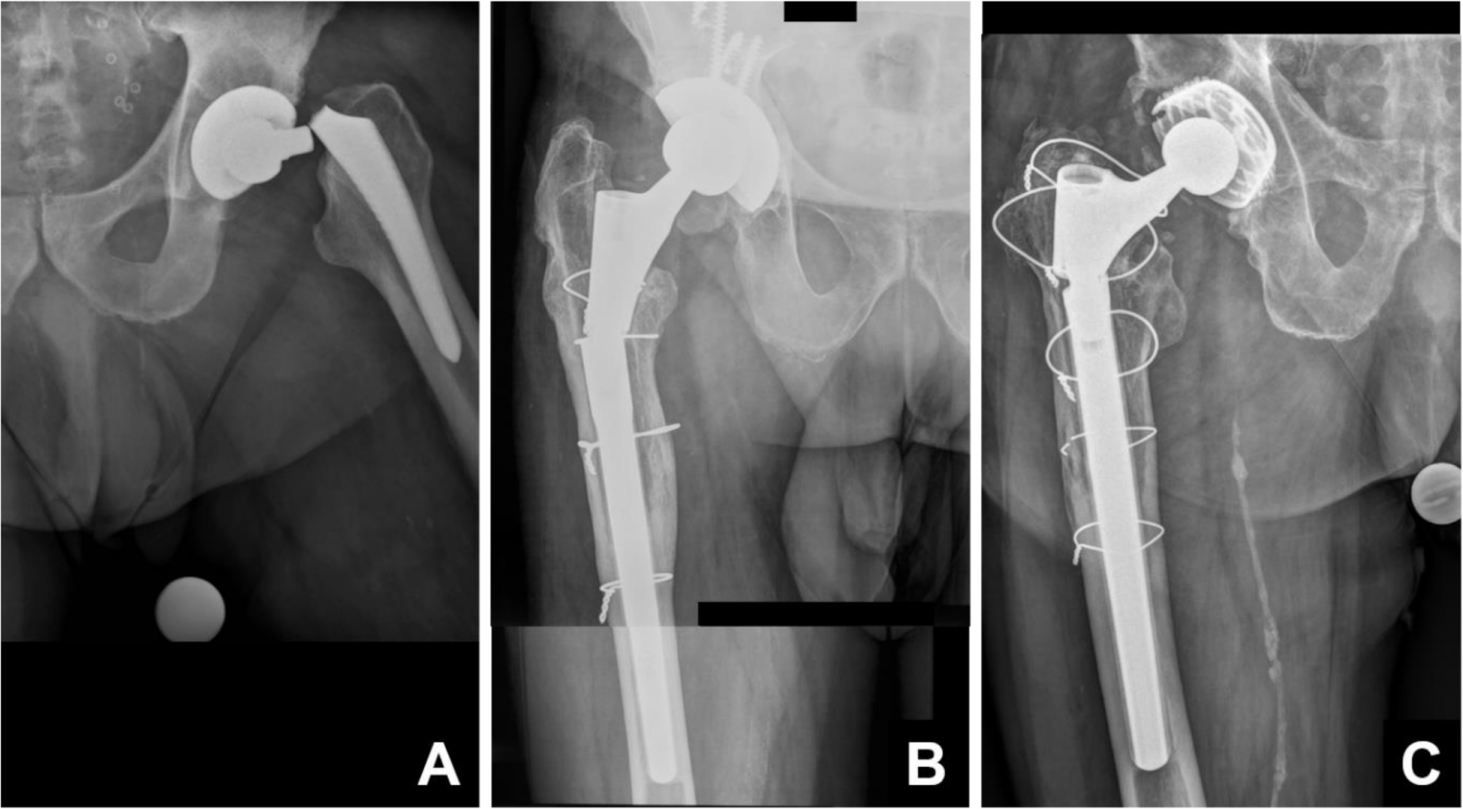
Zone of interest of the anteroposterior pelvic radiographs of three different patients presenting with a well-integrated, broken, uncemented stem after hip arthroplasty. All cases have in common that no standard extraction component can be fitted anymore. In a, a 76-year-old male patient presenting less than 4 years after stem-preserving revision with exchange of the head, performed due to instability, with a well-fixed uncemented primary stem (AMIStem-H, Medacta, Castel San Pietro, Switzerland) broken at the neck. Analysis of the retrievals confirmed electrocautery damage as the cause of the fatigue fracture. In b, a 72-year-old male patient presenting 6 years after stem exchange performed due to a periprosthetic fracture through a transfemoral approach, with reconstruction performed with a tapered, fluted, modular, straight 200-mm stem with distal anchoring (Revitan straight, Zimmer Biomet, Winterthur, Switzerland). Failure was at the level of the connection pin, which had milled out from the bore within the distal component of the stem. In c, a 73-year-old male patient presenting with recurrent failure of the modular connection of a stem implanted 15 years earlier through a transfemoral approach. The proximal component is obviously angulated and loosened again. Reconstruction had been performed with a tapered, fluted, modular, straight 200-mm stem with distal anchoring (PFM-R, Zimmer Biomet, Winterthur, Switzerland) due to aseptic loosening of a previously implanted cemented stem. Nine years later, another revision with exchange of the proximal component due to loosening of the modular connection had been performed
